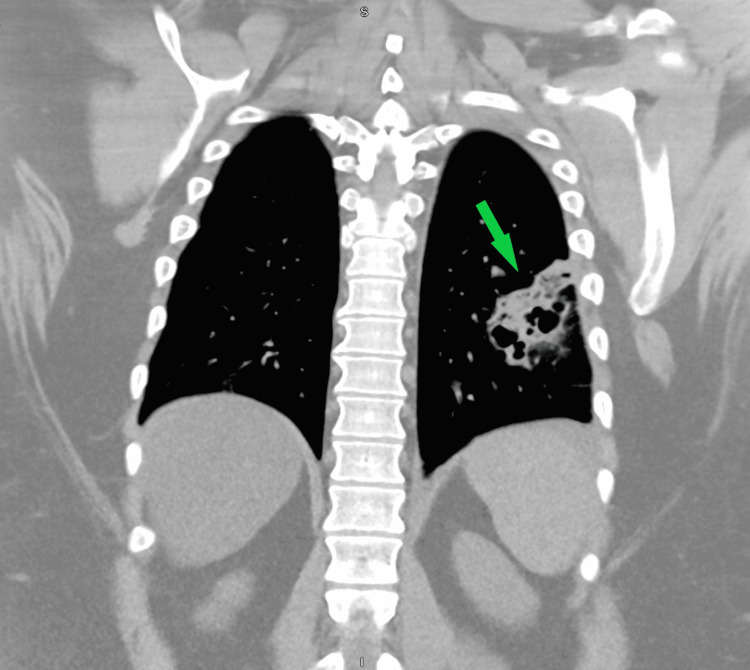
CT of the chest showing multiple cavitary lesions in the left lung consistent with pulmonary septic emboli CT, computed tomography.
